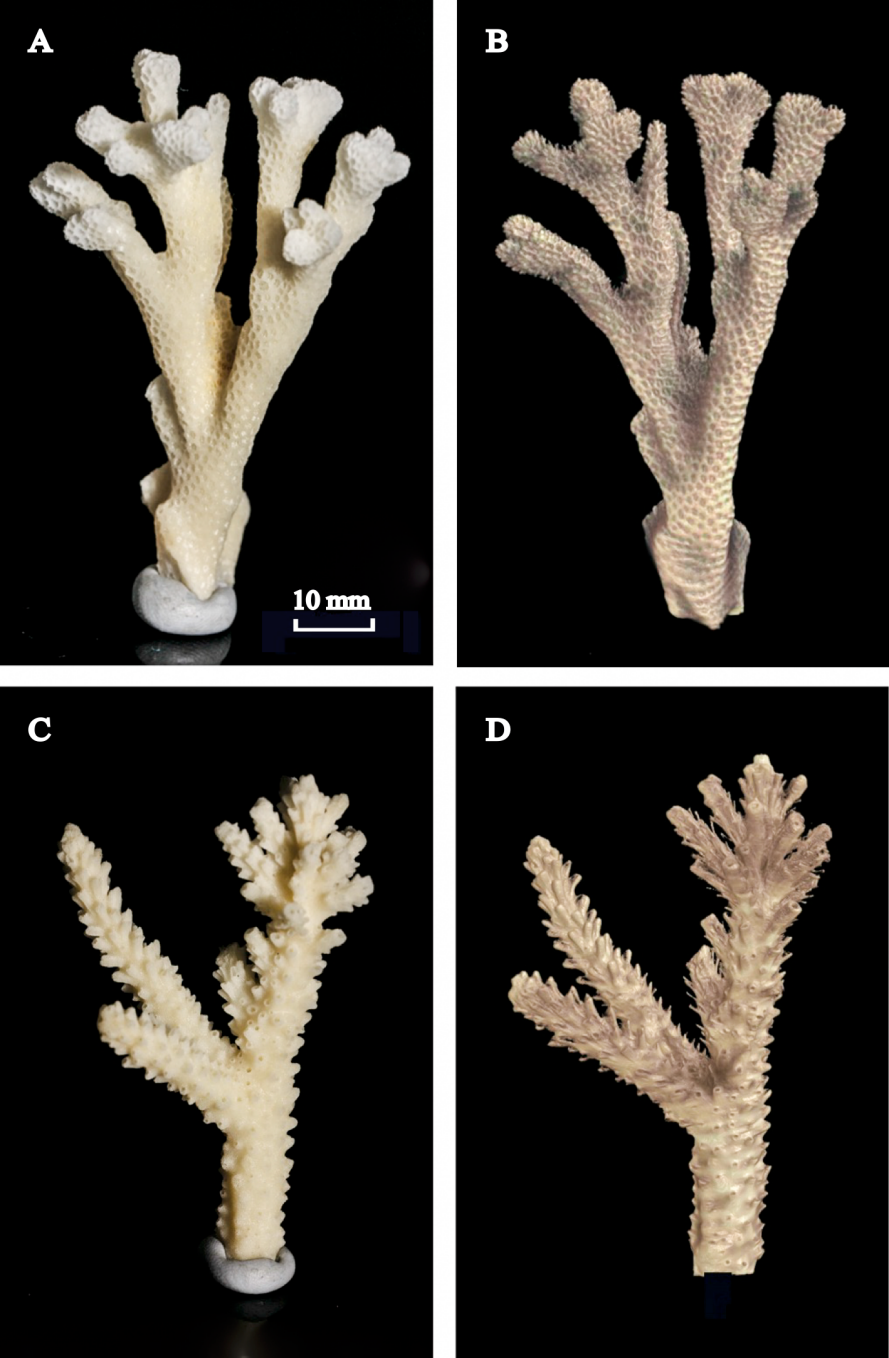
Photographed coral skeletons versus the 3D reconstructions using X-ray CT scans. Photographs of skeletons for (A) *Pocillopora damicornis* and (C) *Acropora yongei* versus (B, D) the digital reconstructions of the same branches using X-ray CT scans. Scale = 10 mm for all images. Photographs by C. Ross (2013).
